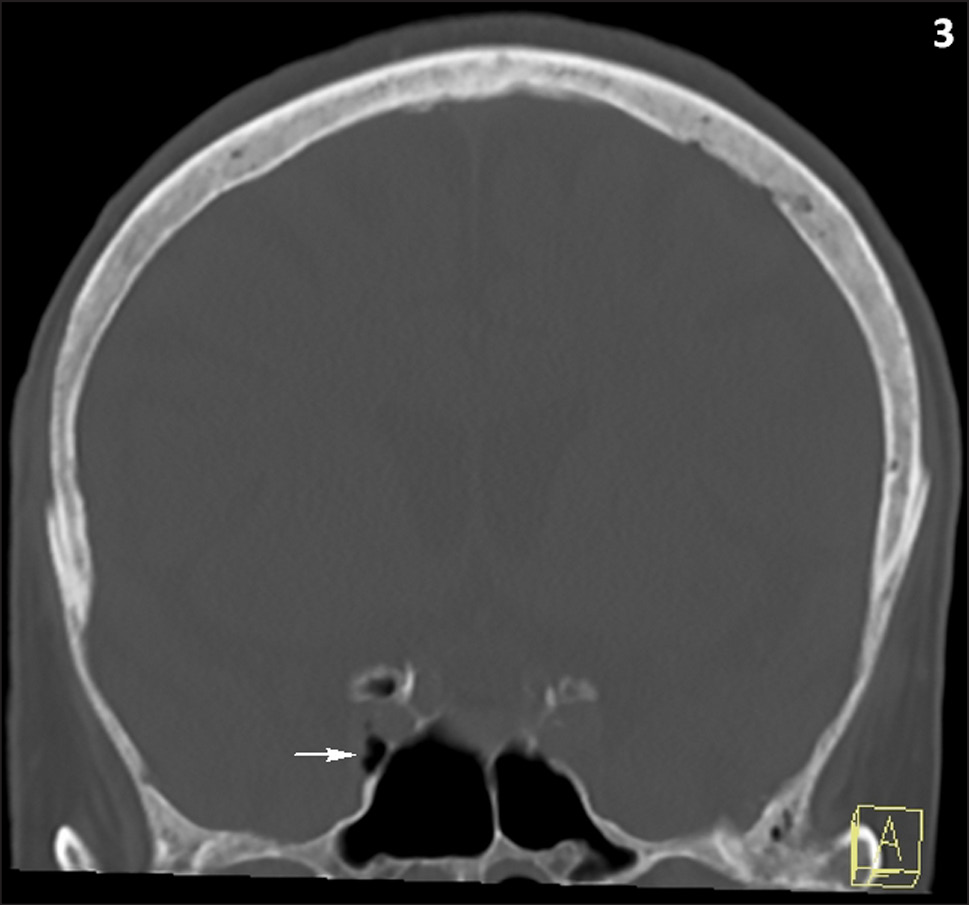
CT of the skull, bone window, coronal reformatted image. Air bubbles at sinus cavernosus (white arrow). Normal pneumatisation of the sphenoid sinuses and absence of a fracture.
